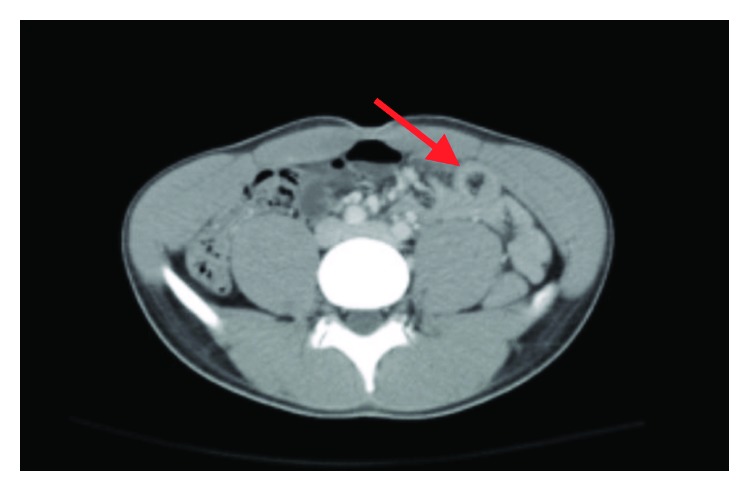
CT of the abdomen and pelvis shows bowel-within-bowel configuration (arrow), where the layers of the bowel are duplicated forming concentric rings.
